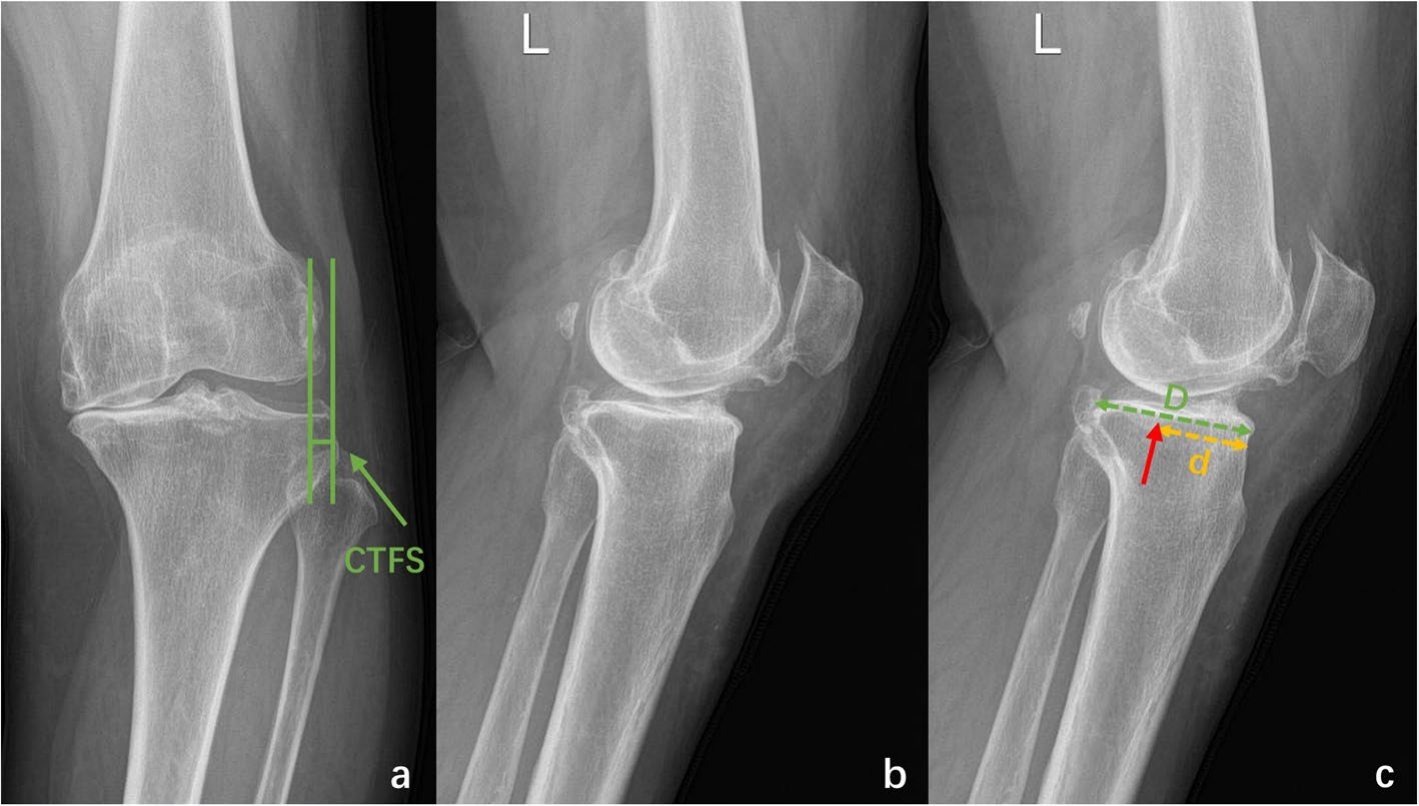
Measurement of Coronal tibiofemoral subluxation (CTFS) and the maximum wear point of the proximal tibia (MWPPT) a-c The anterior–posterior (AP) standing knee X-ray and lateral X-ray of an anterior cruciate ligament-deficient (ACLD) patient (a-c). CTFS is defined as the distance between the tangent line to the outermost joint edge of the lateral condyle of the femur and the tangent line of the lateral tibial plateau (a). The red arrow indicates the maximum wear point of the proximal tibia. MWPPT% is recorded as the ratio between the distance from the maximum wear point to the anterior edge of the tibia (yellow dotted line) and the length of the medial tibial plateau (green dotted line) (c)
